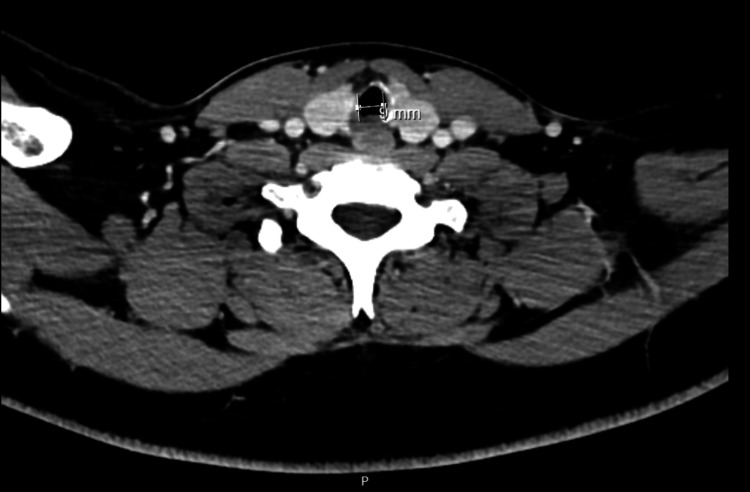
Axial cervicothoracic CT scan image showing a subglottic formation and a minimum diameter of 9 mm in the subglottic larynx
